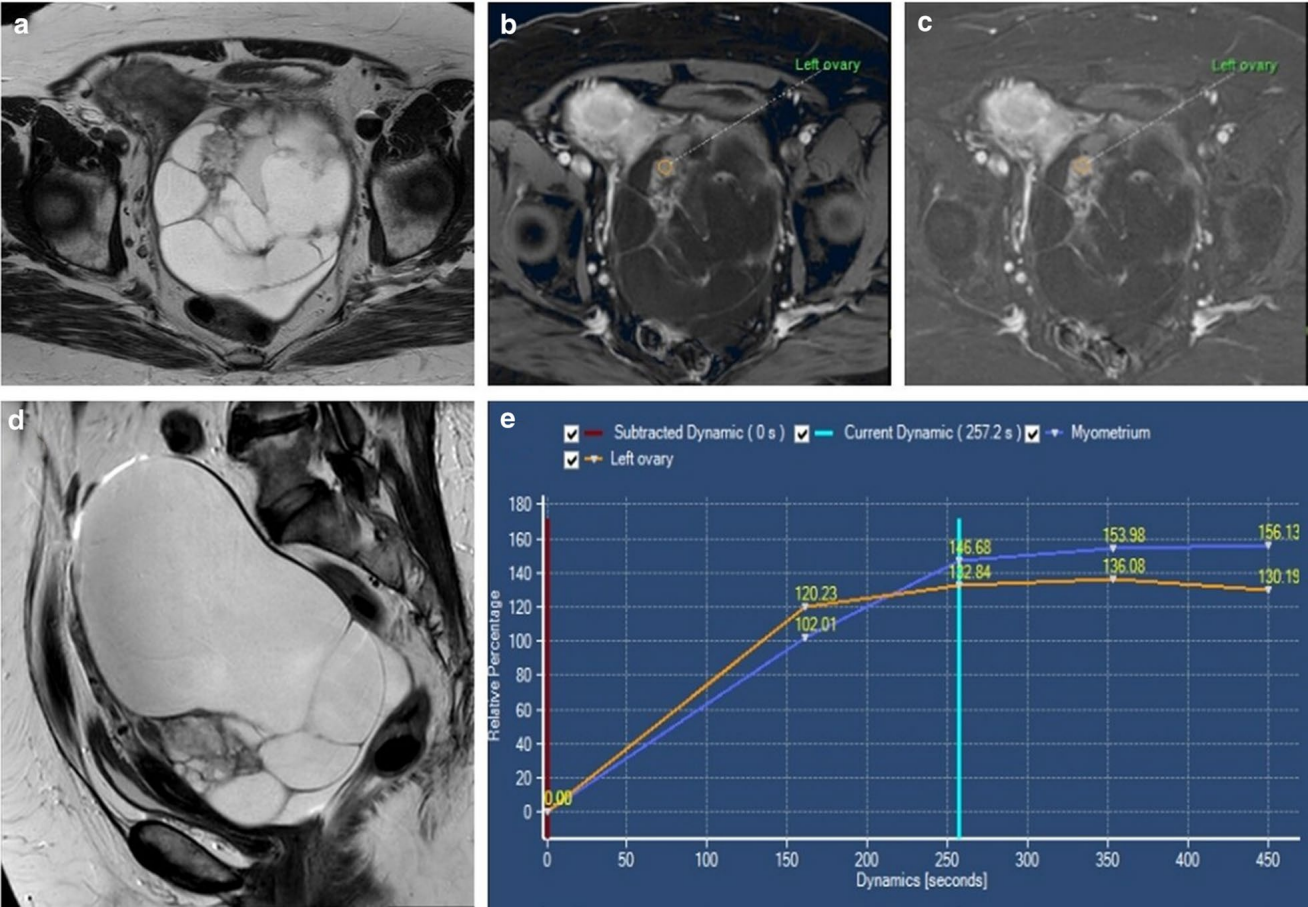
LGSC of the left ovary in a 61-year-old female. Axial and sagittal T2-weighted MR images (a, d), axial T1-weighted MR image with fat saturation, after intravenous gadolinium administration (b) and post-contrast subtraction (c) demonstrate a large multicystic left ovary tumour, with some thick septations and solid parietal nodules. The larger nodule displays a type 3 contrast enhancement curve (e), which is commonly found in malignant epithelial ovarian tumours. It shows an initial contrast uptake higher than the myometrial uptake, followed by washout
